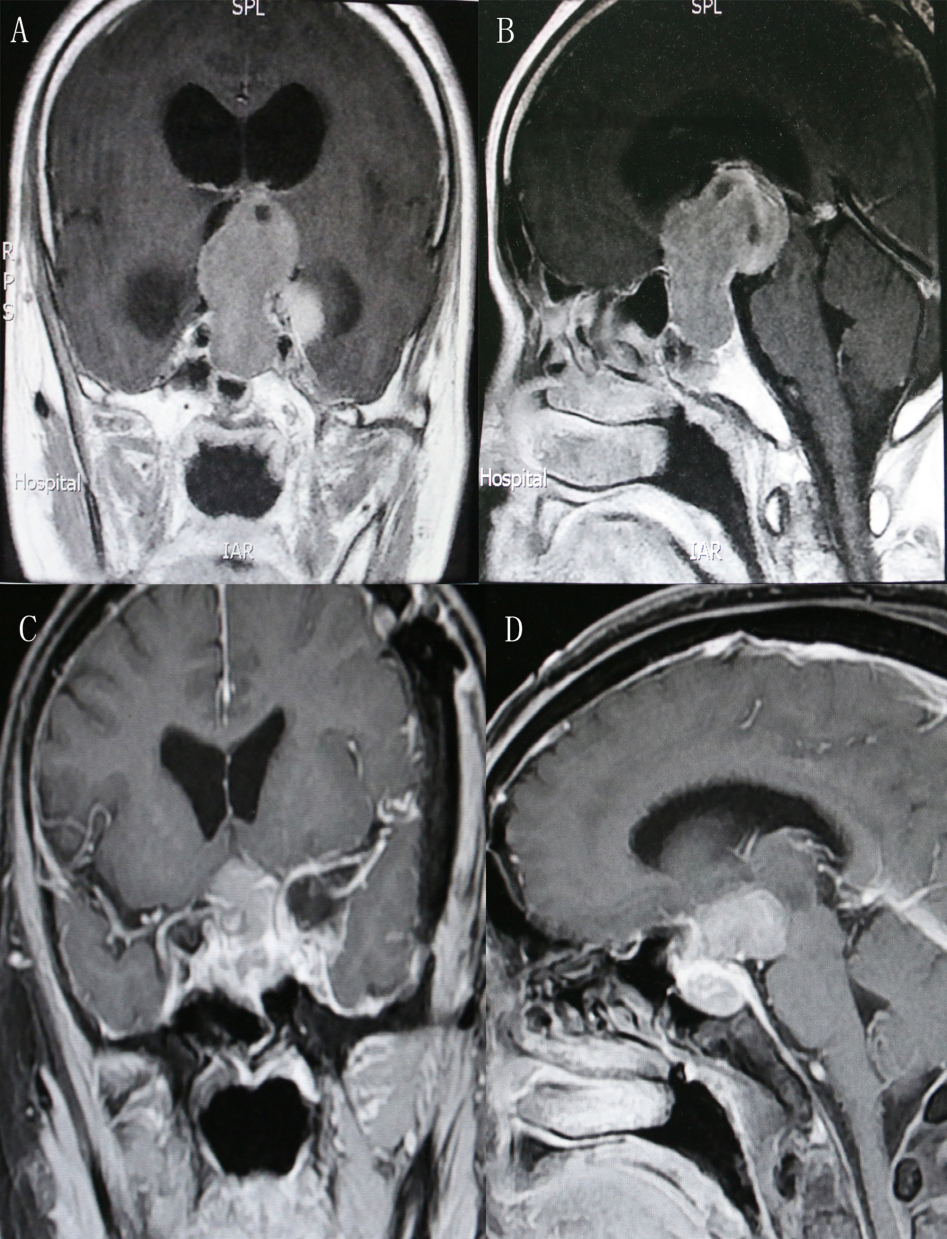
Preoperative coronal (A) and sagittal (B) contrast-enhanced MRI indicated a dumb-bell-shaped adenoma and obstructive hydrocephalus. Postoperative coronal (C) and sagittal (D) view demonstrated a STR of the tumor. MRI, magnetic resonance imaging; STR, subtotal resection.
